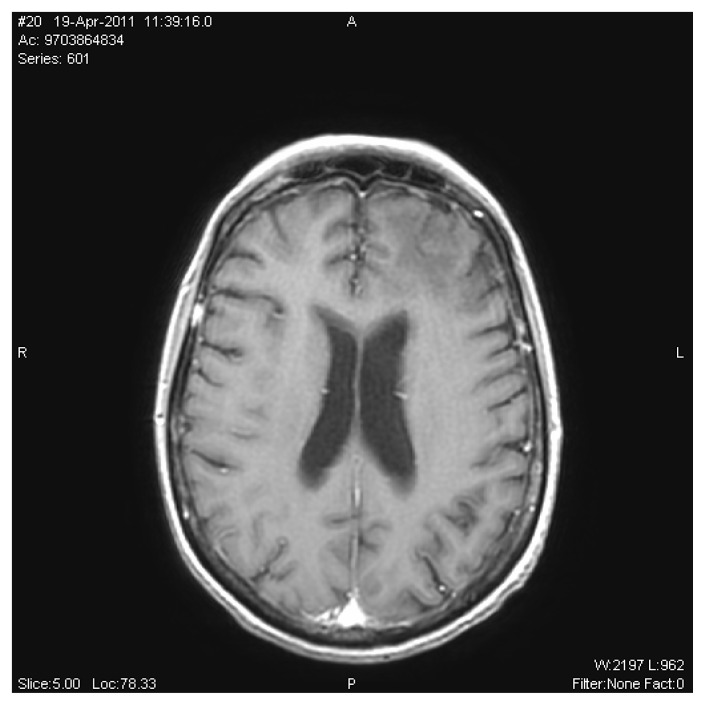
Brain magnetic resonance imaging (MRI) in B1000 diffusion sequence showed a restriction of diffusion in the damage areas of the white matter.
